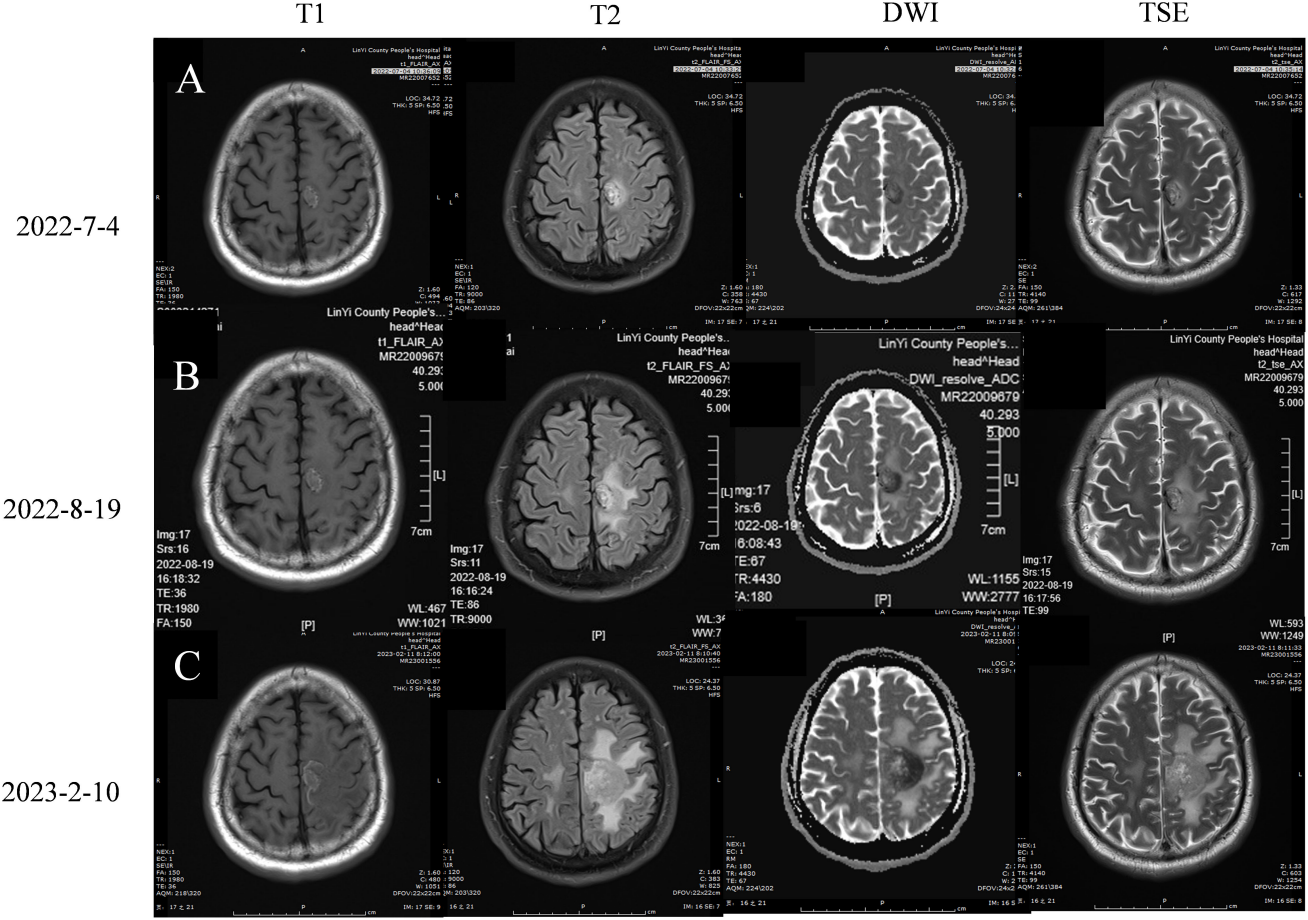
Brain magnetic resonance imaging (A) 2022-7-4 Brain MRI plain scan + enhancement +DWI:On the left parietal lobe, there is a circular lesion with short T1, long T2, and high FLAIR abnormal signals, with clear edges. The DWI shows high and low signals, measuring 1.4 * 1.6cm in size. Localized cortical discontinuity and blurred boundary are observed in the left parietal bone. (B) 2022-8-19 Brain MRI plain scan + DWI+MRA: On the left parietal lobe, there is a circular lesion with short T1, long T2, and high FLAIR abnormal signals, with clear edges. DWI shows high and low signals, with no significant changes compared to the 2022-7-4 image. Localized cortical discontinuity and blurred boundary are observed in the left parietal bone, with no significant changes compared to the previous image. The basilar arteries were in normal shape and the blood flow signal was not abnormal. The wall of the M1 section of the bilateral middle cerebral artery is rough and straight, and the branches below the M2 section are reduced and thin. The blood flow signal was weakened in the anterior part of the left anterior cerebral artery traffic, and the blood flow signal was weakened in the bilateral posterior cerebral artery, which was thin and out of shape. Imaging diagnosis: 1. Multiple ischemic lesions in the brain; 2. The MR results are consistent with left frontal lobe brain metastasis and left parietal bone metastasis. It is consistent with MRA manifestation of atherosclerosis. (C) 2023-2-10 Brain MRI plain scan + enhancement +DWI: In the left frontal parietal lobe, short T1 and long T2 high FLAIR abnormal signal foci were found, such as circular ones, with clear edges and high and low DWI signals. The maximum cross section was about 3.3x3.4cm, which was larger than the range of 2022-8-19, and a long flake T2 edema band was seen around it. The lesion of the left parietal bone showed irregular and mild enhancement, which was larger than that of 2022-8-19.
